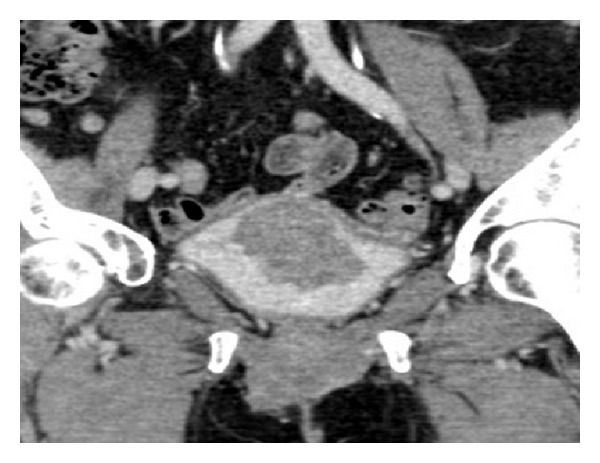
CT urogram (coronal urographic phase image) demonstrates a large polypoid mass arising from the bladder wall. This was confirmed to be a urothelial cell carcinoma following biopsy at cystoscopy.
